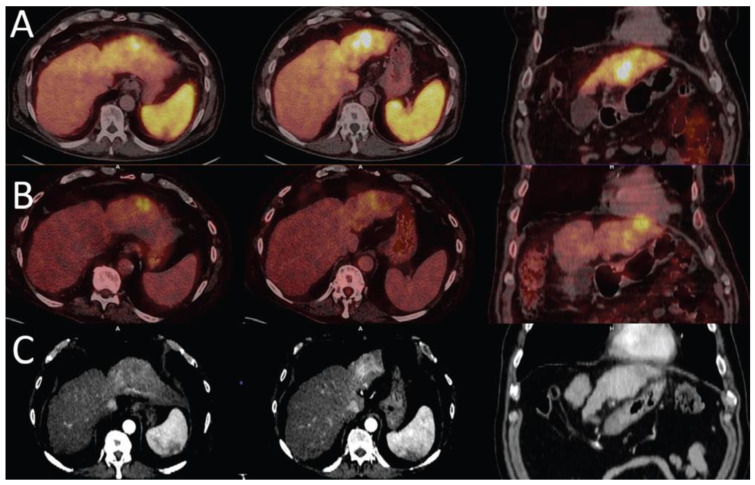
Liver cirrhosis with HCC in the left lobe of the liver. ^68^Ga PSMA (A) shows higher uptake in the hyperenhancement areas of the arterial phase CT images (C) than the focal ^18^F -FDG uptake area (B), which is noted to appear hypodense on CT (images reproduced with permission from [46]).
